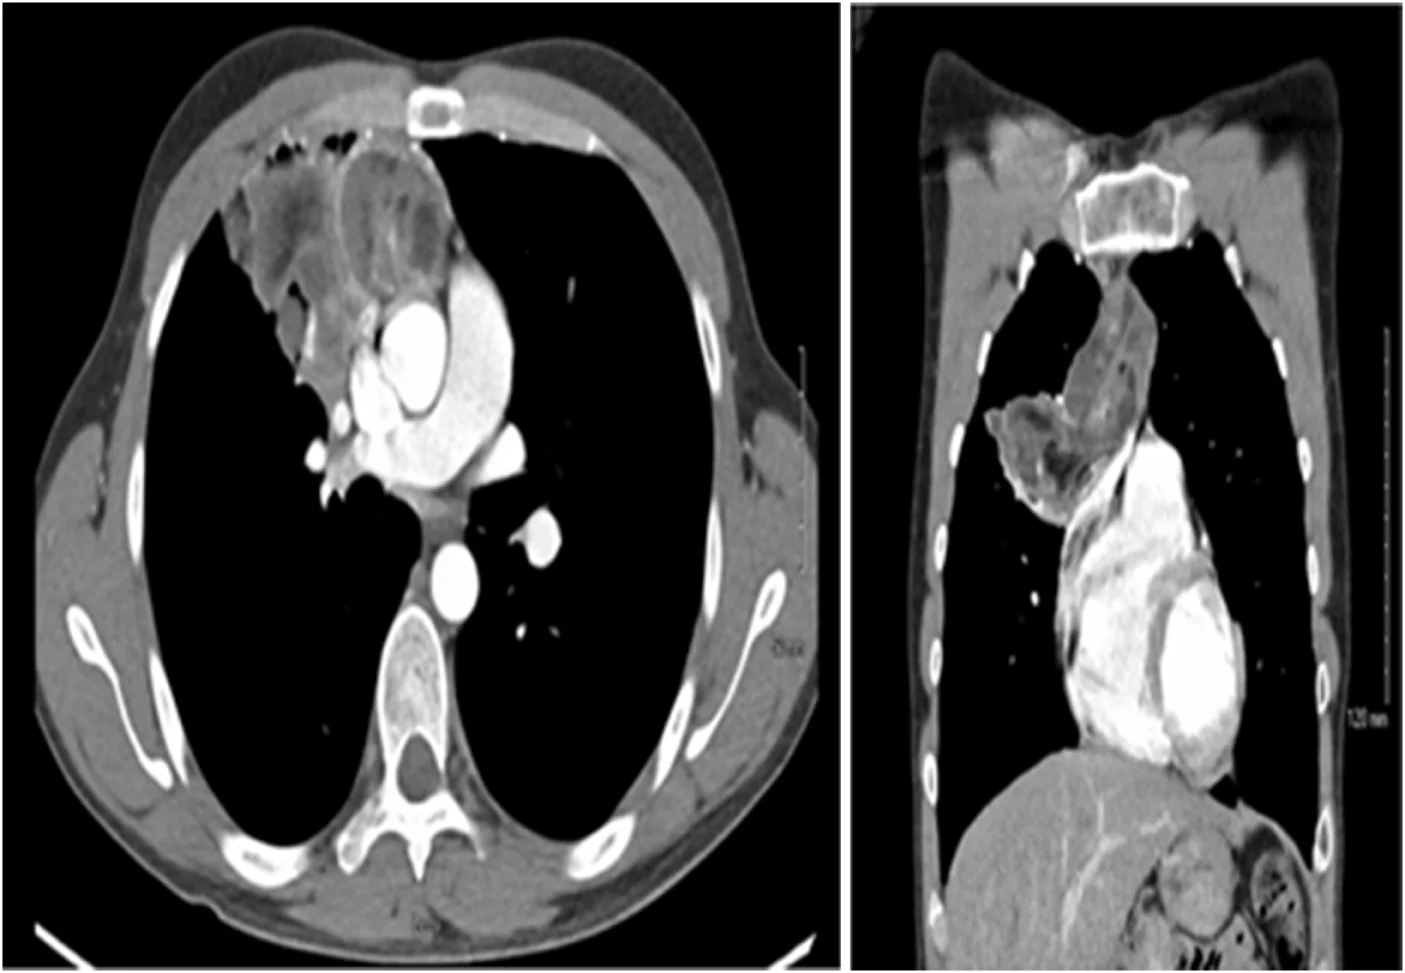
Preoperative chest CT scan showing a 7.9 × 5.8 × 9.7 cm heterogeneous anterior mediastinal mass containing fat, soft-tissue elements, blood vessels, and calcification in axial and coronal sections.
